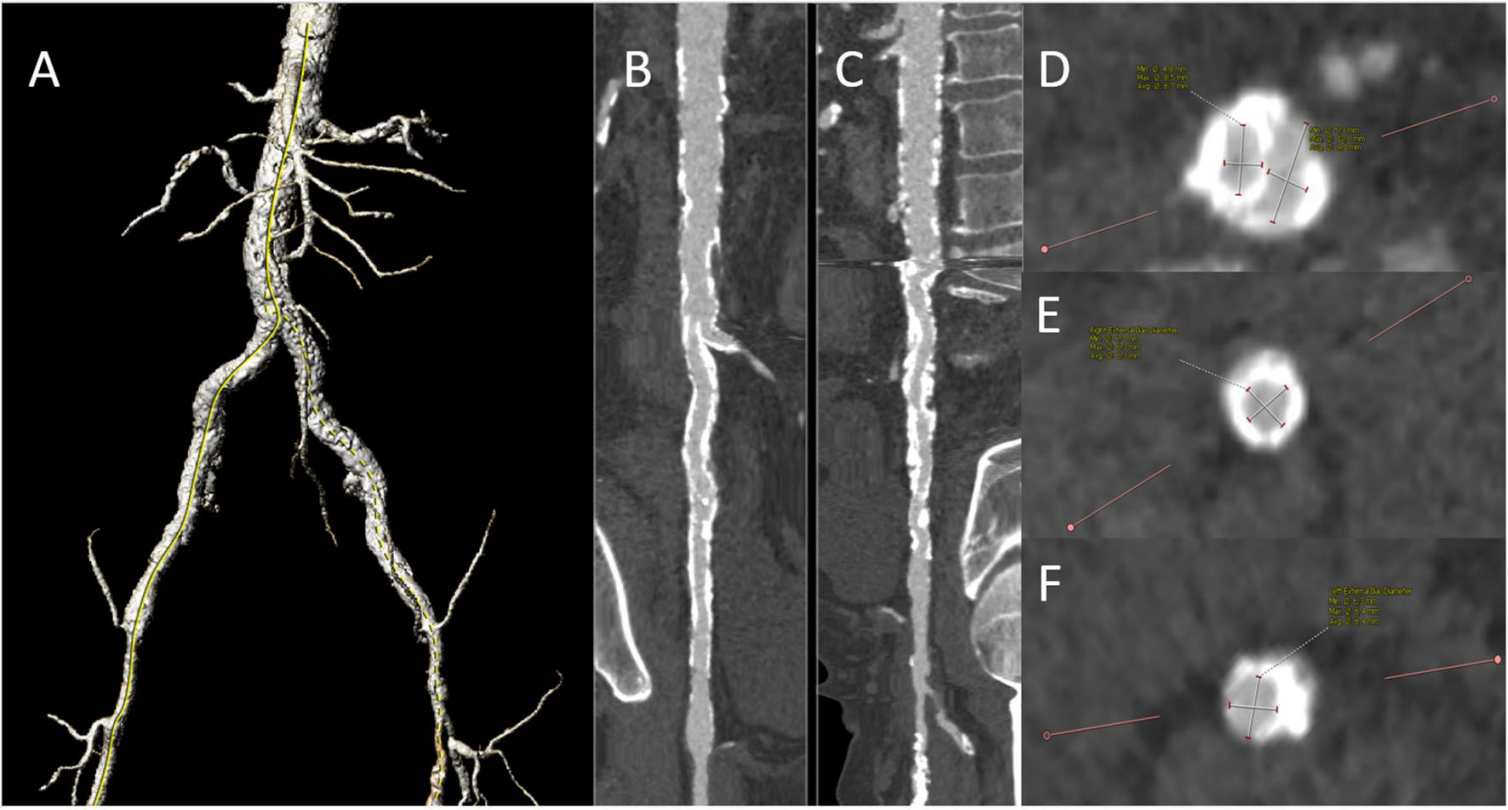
Preinterventional CT angiography demonstrating severe aorto-iliac PAD: 3-D reconstruction of pelvic vessels (A). A stretched vessel analysis of the right (B) and left (C) axis and infrarenal aorta demonstrating severe calcification. Slice of the iliac bifurcation (D), the right external iliac artery (E), and the left external iliac artery (F) Both the iliacs were already provided with the self-expandable stents in the past. Duplex ultrasound displayed hemodynamically severe iliac stenoses with a peak systolic velocity of 2.6 m/s in the right common iliac and 3.0 m/s in the left external iliac artery. We decided to perform a staged procedure with the preparation of the iliac vessels first.
